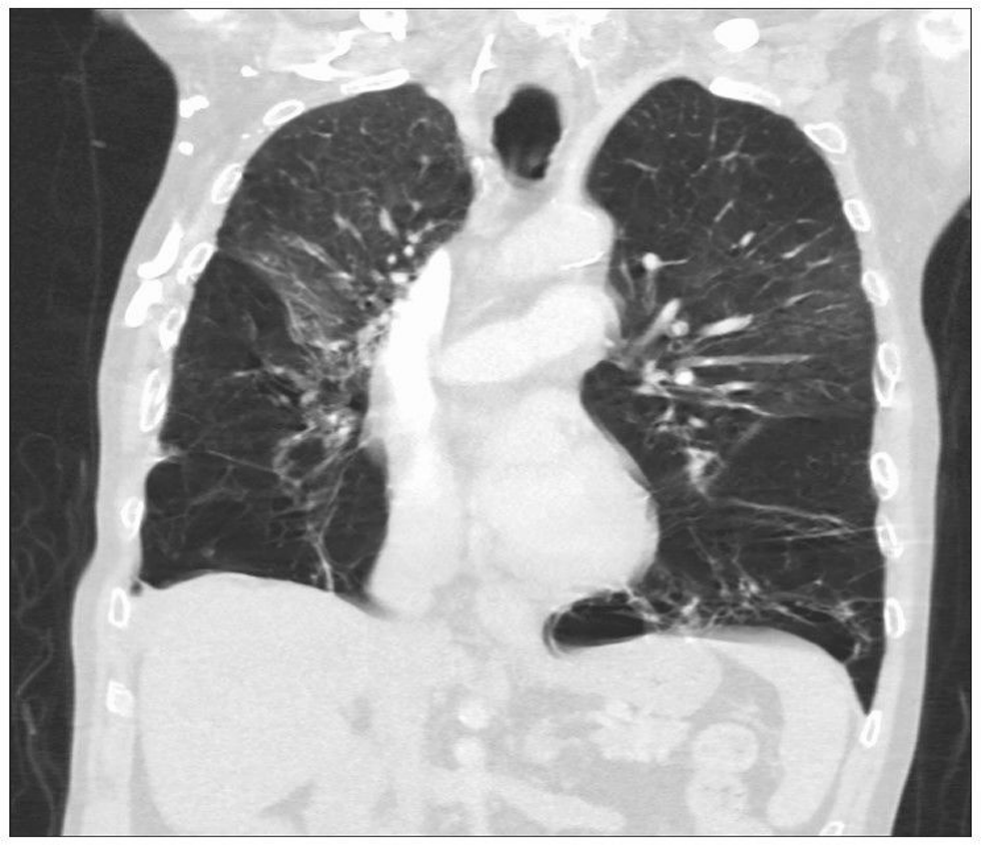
A computed tomography scan showing severe bilateral, lower lobe-predominant panacinar emphysema in a patient with AATD homozygous for the Z mutation. Abbreviation: AATD, alpha-1 antitrypsin deficiency.
